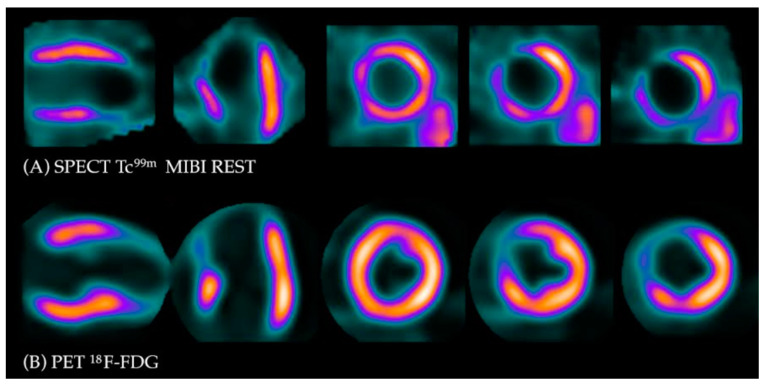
Myocardial viability imaging: Match pattern, non-viable scarring. (A) Myocardial perfusion imaging with MIBI-Tc^99m^ SPECT: heart scans showing lack of tracer uptake in the apical region of the left ventricle (LAD territory). (B) ^18^FDG viability PET. ^18^FDG uptake is absent in the area of the perfusion defect, confirming myocardial scarring in this region.
